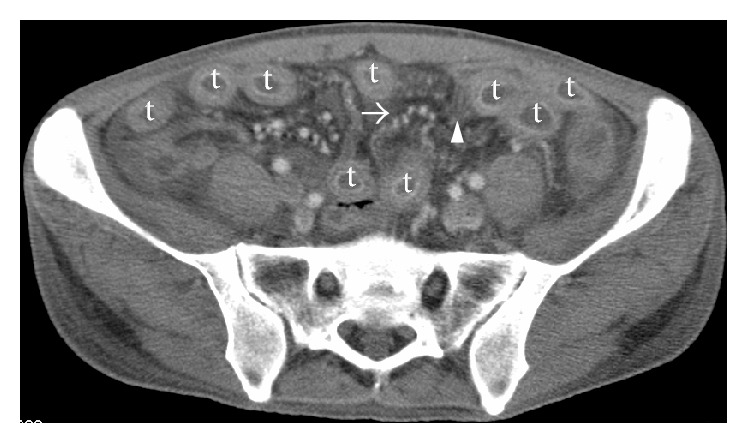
CT scan reveals bowel wall thickening and enhancement and engorgement of mesenteric vessels. Target sign (t), comb sign (arrow head), and nodular sign (arrow).
